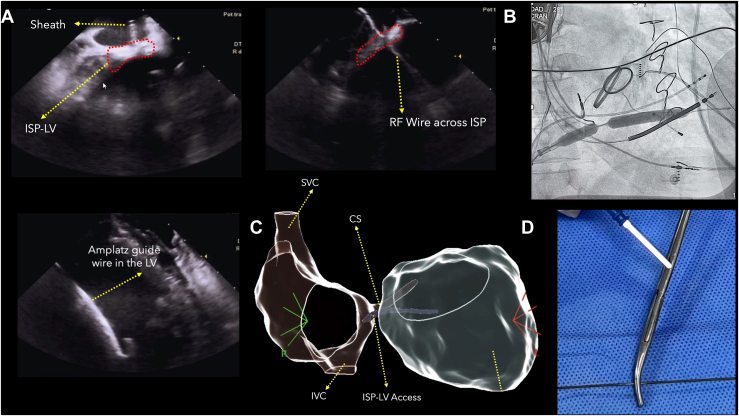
A: Intracardiac echocardiography (ICE) showing the deflectable sheath positioned in the right atrium (RA) and directed toward the inferoseptal process of the left ventricle (ISP-LV) (left) as well as a successful radiofrequency (RF) wire crossing into the LV (right). B: Fluoroscopic right anterior oblique projection demonstrating inflation of a 9-mm noncompliant balloon at the RA-to-LV puncture site to dilate the tract. C: CARTO 3-dimensional electroanatomic map showing RA anatomy and the ISP-LV access site, near the inferior third of the coronary sinus (CS) ostium. D: Custom-modified RF wire with an exposed distal segment (metallic), designed to conduct energy precisely across the septum under ICE and fluoroscopic guidance. IVC = inferior vena cava; SVC = superior vena cava.2.*RA-LV puncture:* Specifically designed radiofrequency wires are not commercially available in our country; instead, a 0.035-in guidewire (Amplatz, Cook Medical) was introduced through the sheath with the stiff tip at the end. The surface metal of a small portion at the proximal end of the wire was exposed to allow contact with electrocautery. Then, the wire was advanced while applying energy (≈25 W for ∼15 seconds), perforating the ISP-LV. Successful LV access was visualized on ICE and fluoroscopy.3.*Wire exchange:* Upon gaining access, a 4-F hydrophilic microcatheter (Terumo GlideCath) was passed over the radiofrequency wire into the LV. Through this microcatheter, the radiofrequency wire was exchanged for a stiff 0.035-in Amplatz Super Stiff guidewire. The Amplatz wire was positioned securely in the LV apex, providing a rail for dilation and sheath advancement.4.*Tract dilation and sheath insertion:* Over the Amplatz wire, a noncompliant angioplasty balloon (9 mm diameter × 40 mm length) was advanced and 2 serial dilations were performed. Then, the Vizigo sheath was advanced over the balloon.5.*Mapping and ablation:* High-density electroanatomic mapping was performed using a mapping catheter (PentaRay, Biosense Webster). Programmed electrical stimulation from the right ventricular apex did not induce any VT under general anesthesia. Therefore, a substrate-based ablation approach was pursued. Voltage mapping in sinus rhythm revealed an extensive inferolateral LV scar, spanning the basal to mid-inferolateral wall. Within the scar, late potentials and fractionated electrograms were identified. Catheter ablation was performed using an irrigated contact force catheter (ThermoCool SmartTouch SF, Biosense Webster), and multiple radiofrequency ablation lesions (45–50 W, temperature-controlled) targeting these abnormal potentials were performed. Programmed stimulation was then repeated from the right ventricular apex, and no sustained VT could be induced postablation. The total procedure time was approximately 4.5 hours, with about 50 minutes required to obtain LV access.
